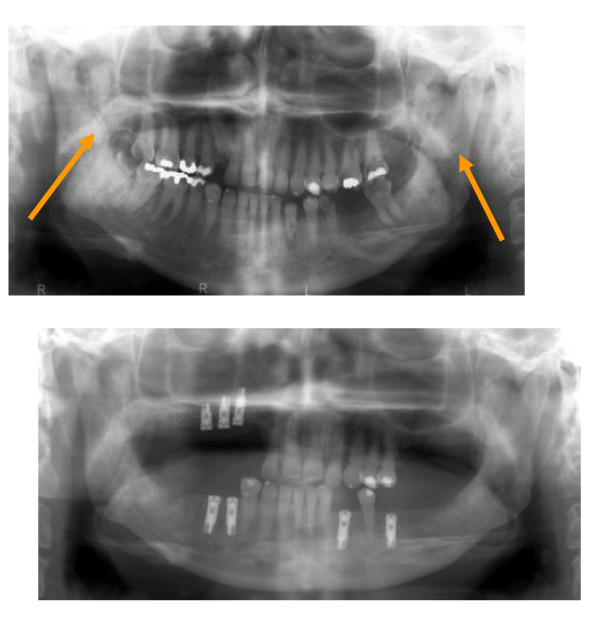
a. Panoramic radiograph with radiopaque mass (arrow). b. After insertion of implants
